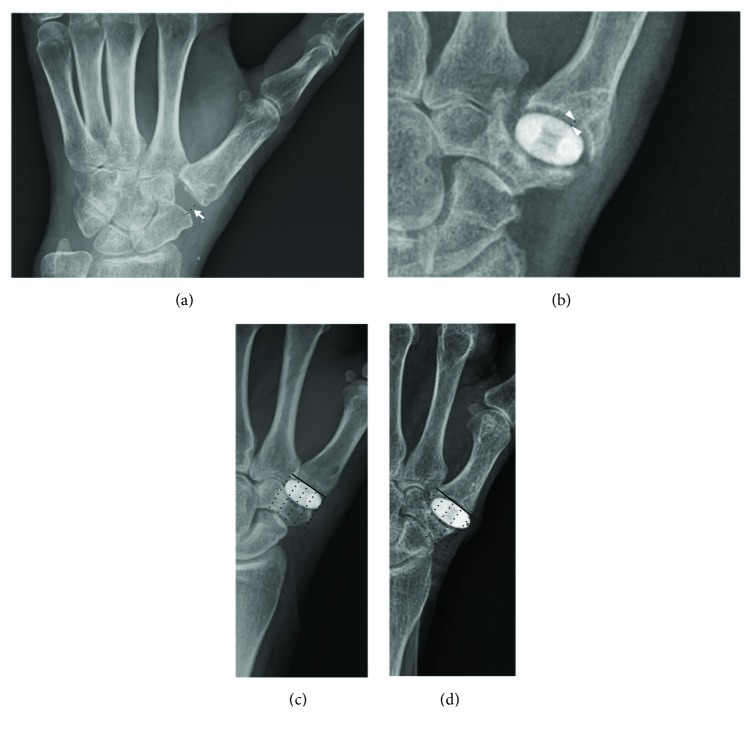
Postoperative radiographic measurements. (a) Proximal migration of the first metacarpal bone (arrow) was measured as the distance between the distal articular surface of the scaphoid and the proximal articular surface of the first metacarpal bone. (b) Radiolucency around the implant (arrowhead) was measured between the edge of the implant and the most distant rim of the surrounding metacarpal bone or trapezium. (c) Implant migration (*∗*) was measured based on divided quarters of the trapeziometacarpal joint and classified as centered, one-fourth displaced, one-half displaced, or greater than one-half displaced.
